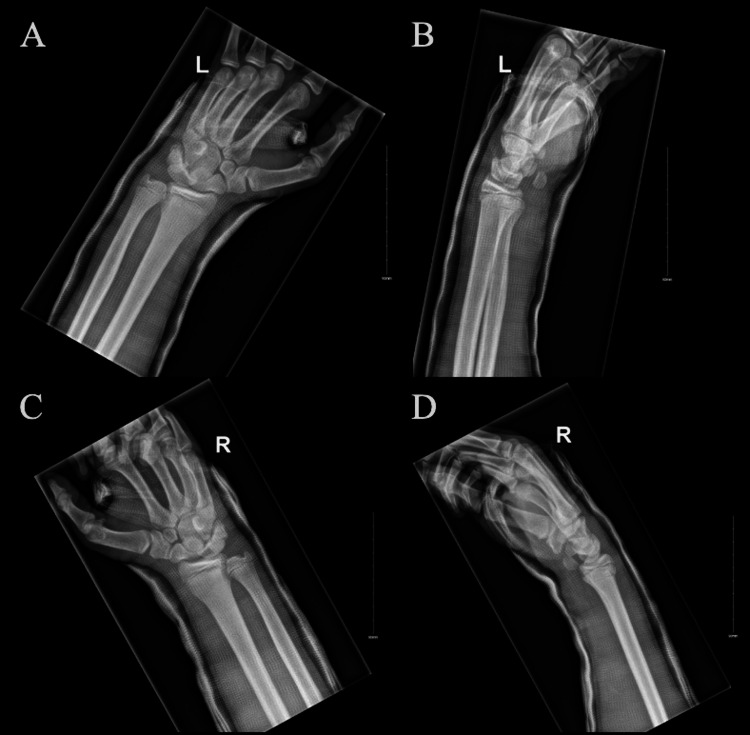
Bilateral wrist radiographs post-reduction Post-closed reduction and short arm casting of bilateral wrists; Posteroanterior (A) and lateral (B) radiographs of the left wrist and posteroanterior (C) and lateral (D) radiographs of the right wrist
